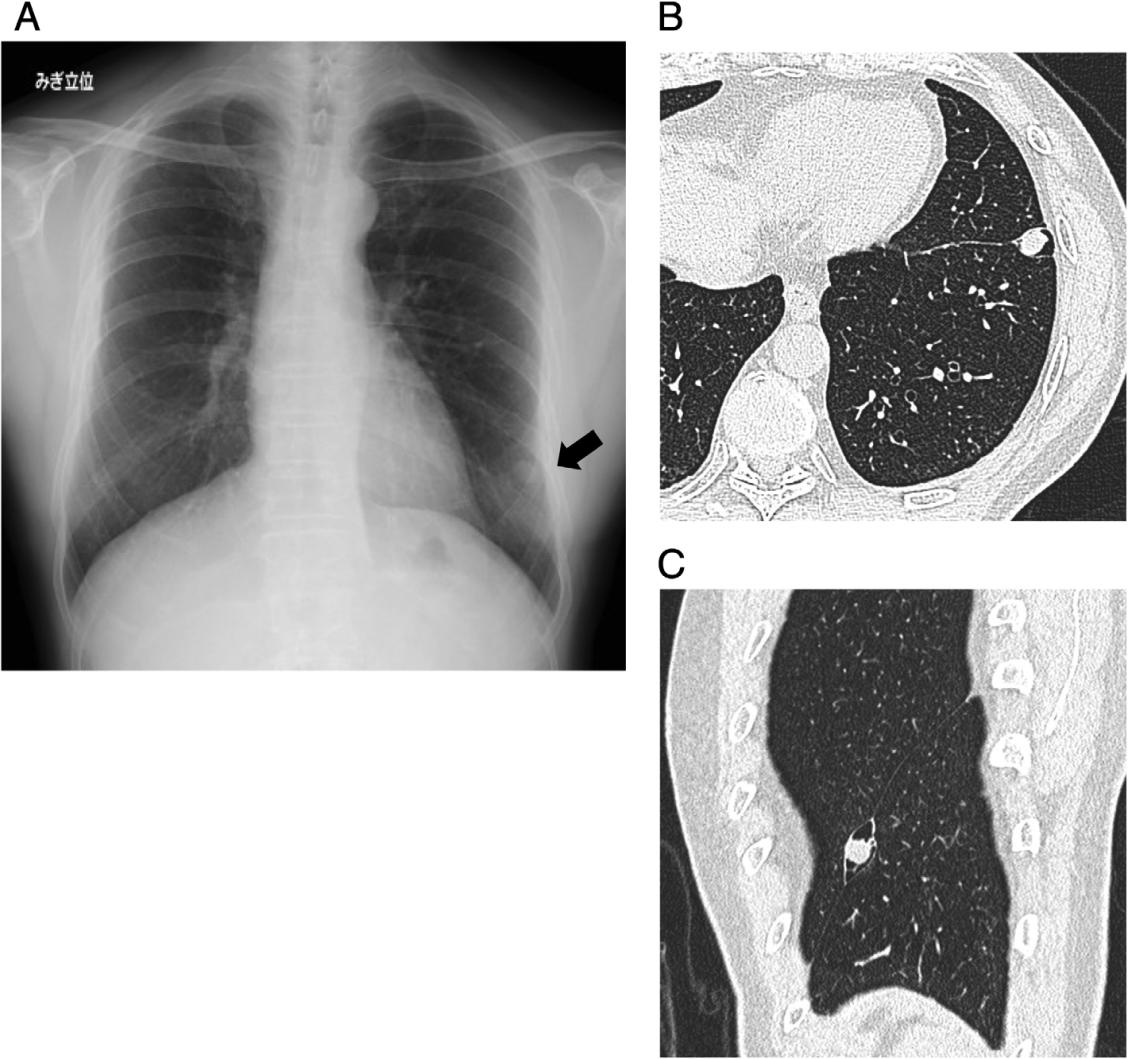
(A) The chest X‐ray showed a well‐defined 15 mm × 11 mm nodule in the left lower lung field. (B, C) Computed tomography (CT) shows a tumour arising in a cavity located in the interlobar space.
